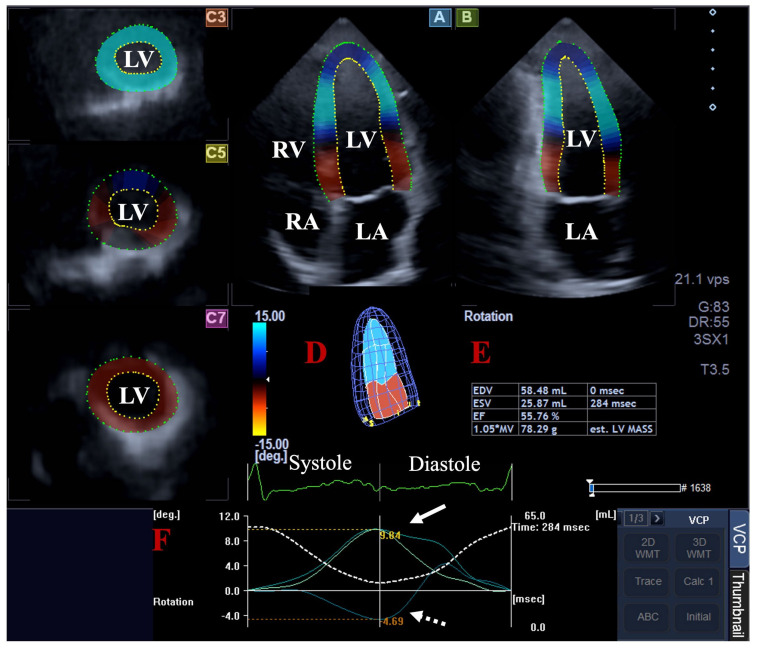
Assessment of left ventricular (LV) rotational parameters by three-dimensional (3D) speckle-tracking echocardiography. Apical four-chamber (A) and two-chamber (B) long-axis views and short-axis views at basal (C3), midventricular (C5) and apical LV levels (C7) are shown with virtual 3D model of LV (D) and calculated LV volumetric data (E). Time-apical (white arrow) and basal (white dashed arrow) LV rotational curves are presented together with a curve representing time–LV volume changes (dashed white line) during heart cycle (F). Abbreviations: LA = left atrium; LV = left ventricle; RA = right atrium; RV = right ventricle; EDV = end-diastolic volume; ESV = end-systolic volume; EF = ejection fraction.
